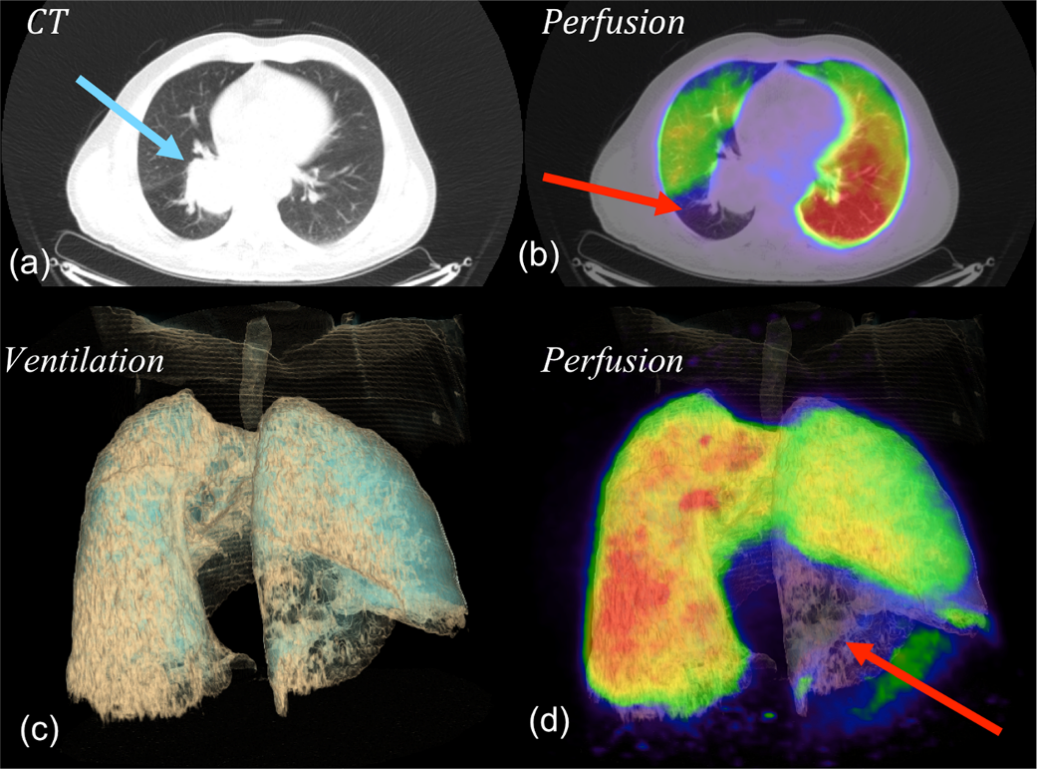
V/Q PET/CT, a) CT alone, b) fused PET/CT, c) 3D volume rendered (VR) CT ventilation reconstruction d) 3D fused VR perfusion PET/CT. A patient with a large upper lobe NSCLC (
